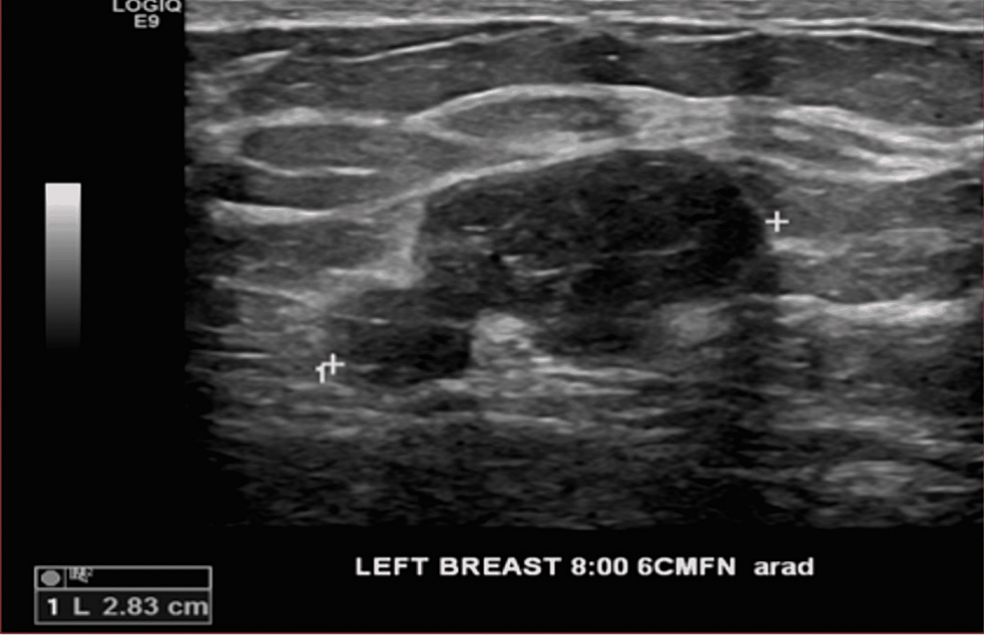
Ultrasound 32 months later demonstrates a hypoechoic 2.8 cm mass that has increased in size, with heterogeneity and lobulations.
